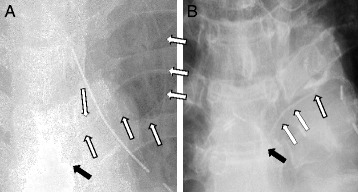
Chest radiographs showing the position of the catheter after insertion and the retained catheter fragment. The *left* image (a) shows the paravertebral catheter after the insertion procedure (*white arrows*). The right image (b) shows the catheter fragment (*white arrows*) remaining after catheter removal. In both images, the *black arrow* indicates the tip of the catheter
